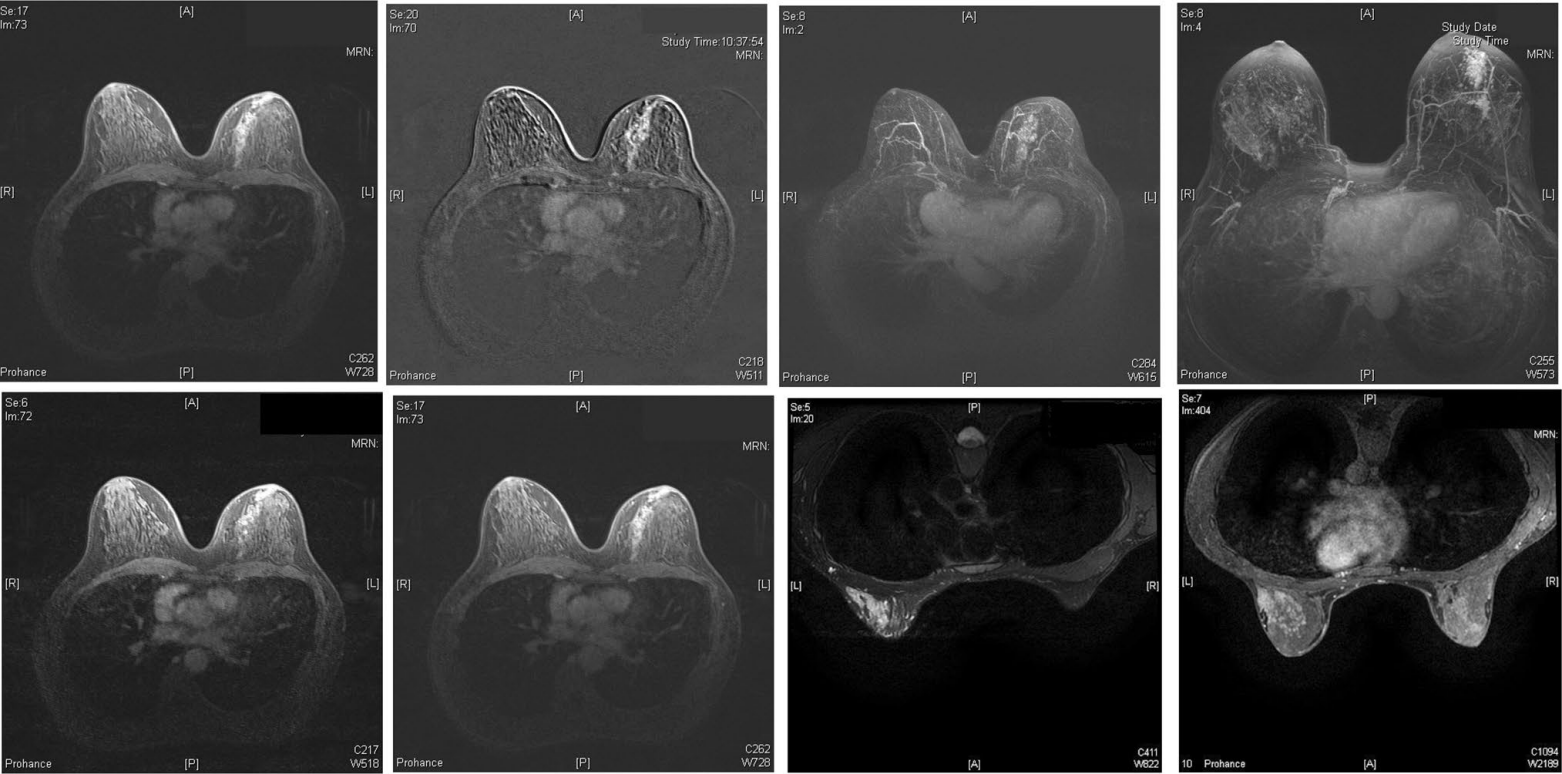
Breast MRIs demonstrating enhancement with ductal/segmental distribution consistent with DCIS in four patients with PND and normal mammography and breast ultrasound
